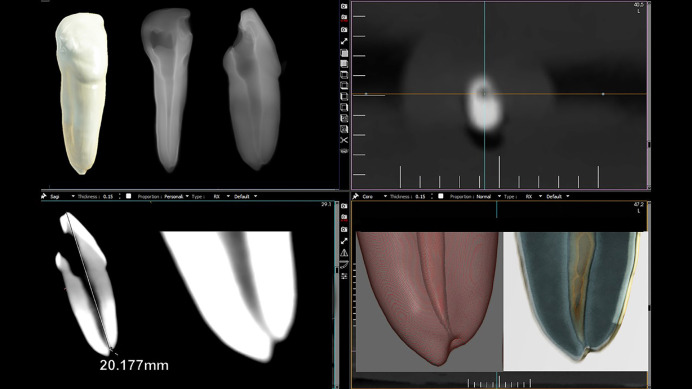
(A-D) CBCT scan with cinematic rendering of a mandibular central incisor, emphasizing the apical region, apical constriction, and apical foramen, along with measurement using the e-Vol DX software.
